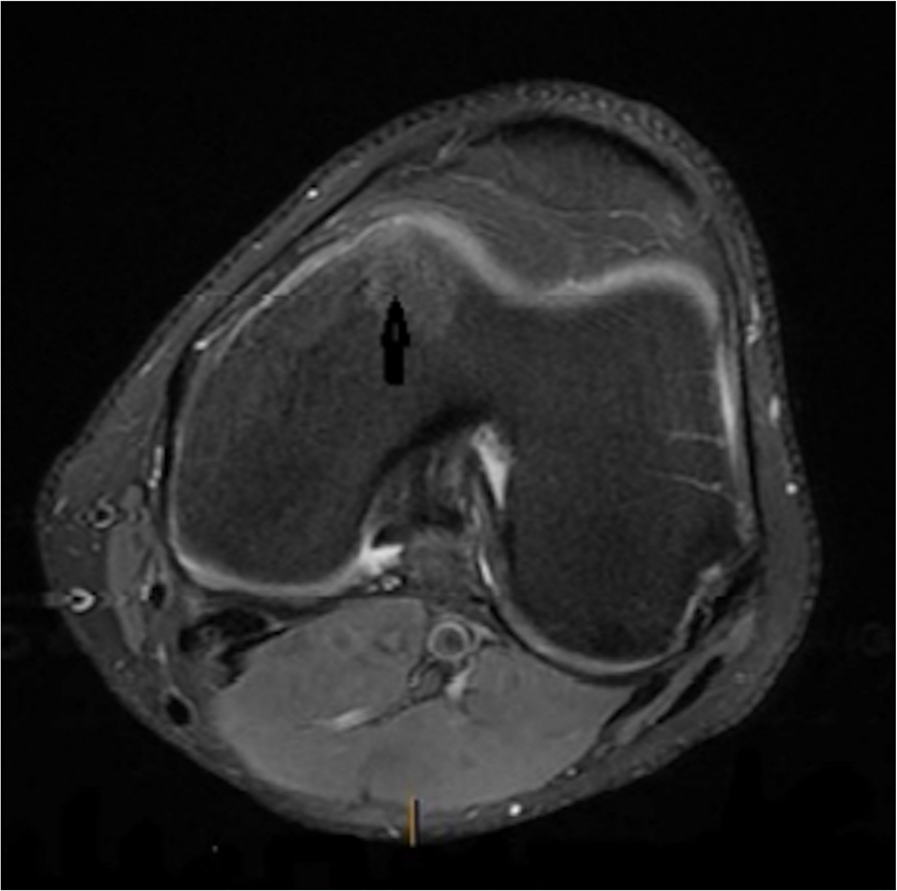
Axial MRI of the left knee showing oedema in the medial femoral condyle
